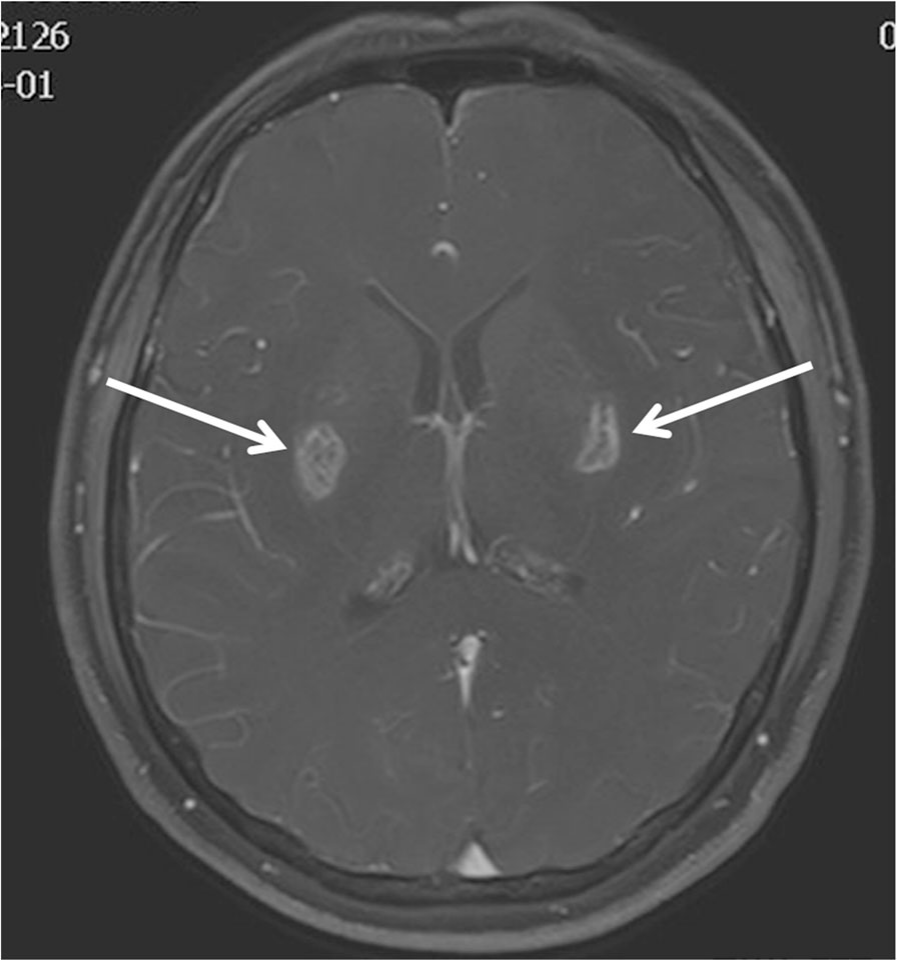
On day 28, magnetic resonance imaging (MRI) after gadolinium was administered revealed abnormal enhancement within the bilateral basal ganglia, and the size of the lesions decreased on MRI conducted 25 days after admission
